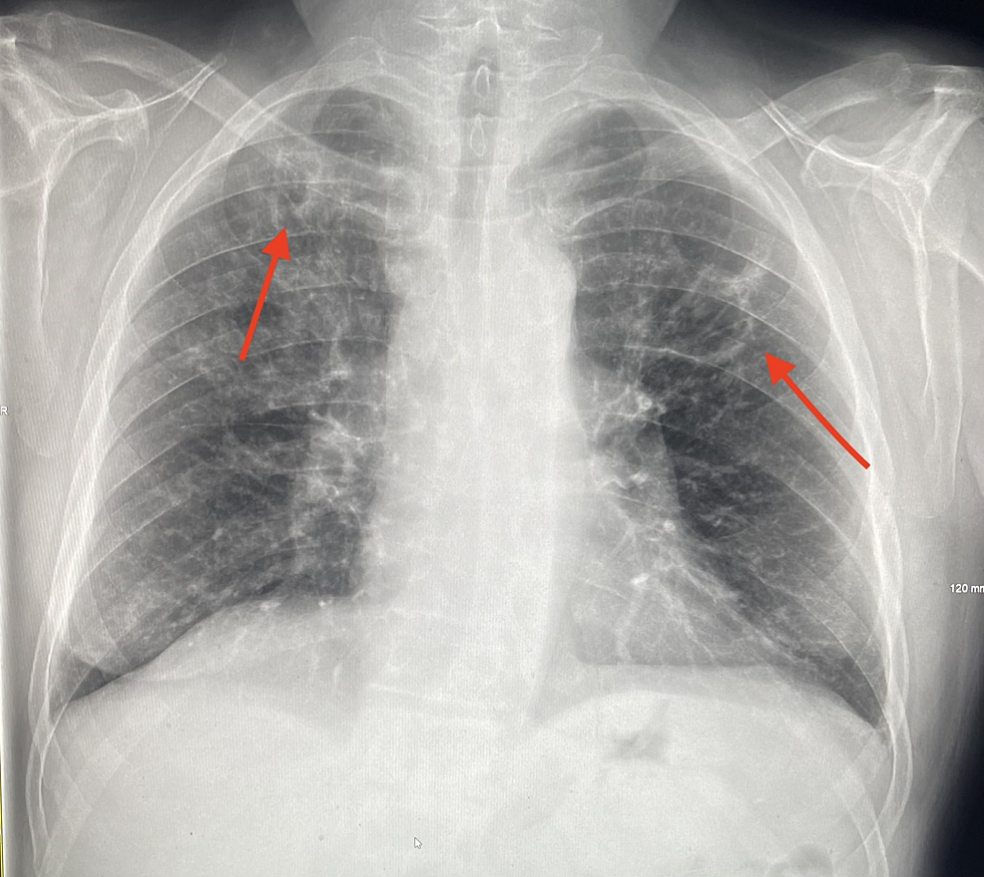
Chest X-ray post-steroid taper showing residual areas of linear opacification of the lungs, within lung apices, suggesting areas of scarring.
